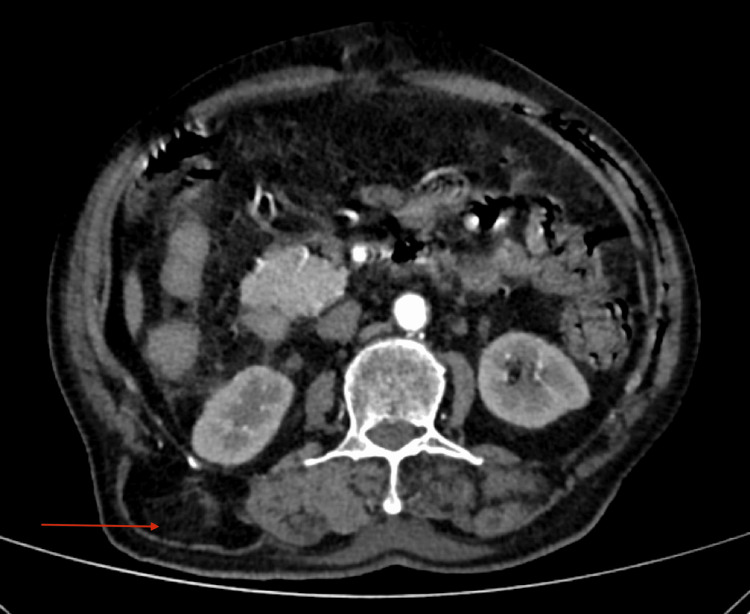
CT of the abdomen showing defect in the right posterior lumbar region
